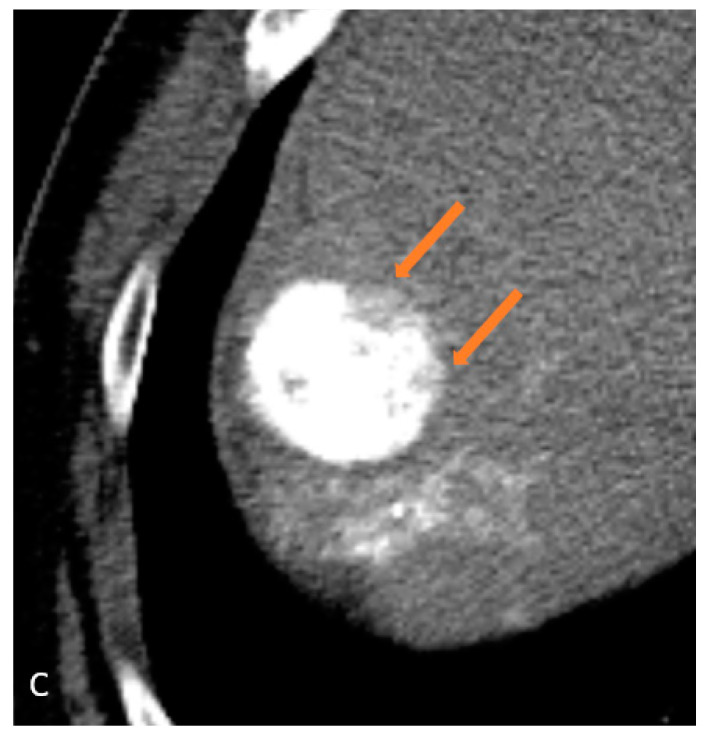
A 63-year-old male with hepatitis C-induced cirrhosis. (A) CEUS demonstrates two areas of focal peripheral nodular enhancement in the treated lesion (blue arrows), indicating residual disease. Initial follow-up CT (B) was read as non-viable lesions (LR-treated). Three-month follow-up CT confirmed CEUS findings of residual disease. Retrospective review of the initial CT demonstrated nodular regions of enhancement, better seen on enlarged view of the lesion ((C), orange arrows).
